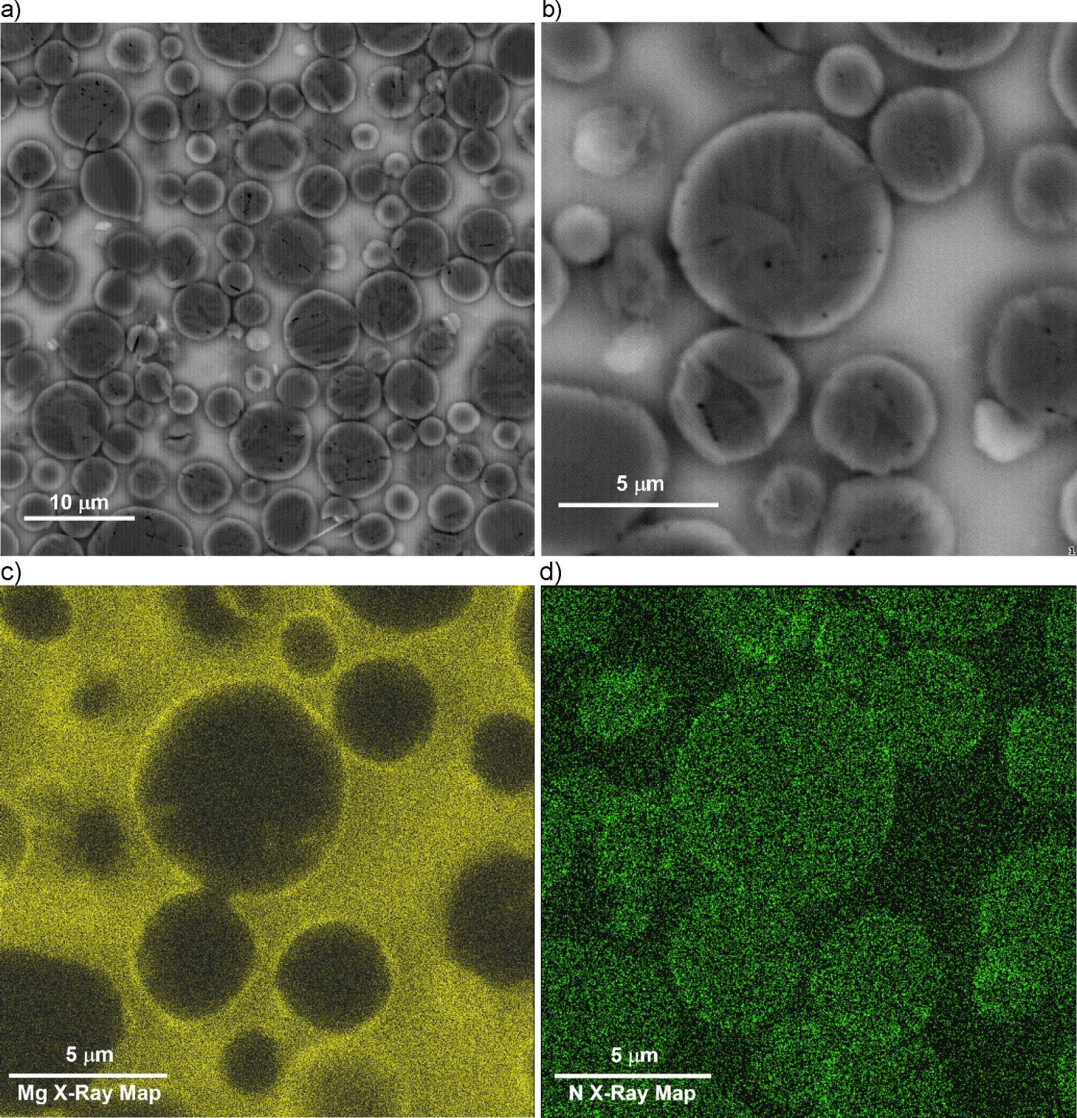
Microstructure within the infill region of an 80% infill density AMC sample. (a) BSE image; (b) high magnification region used for EDS; (c) Mg characteristic X-ray map; (d) N characteristic X-ray map.
